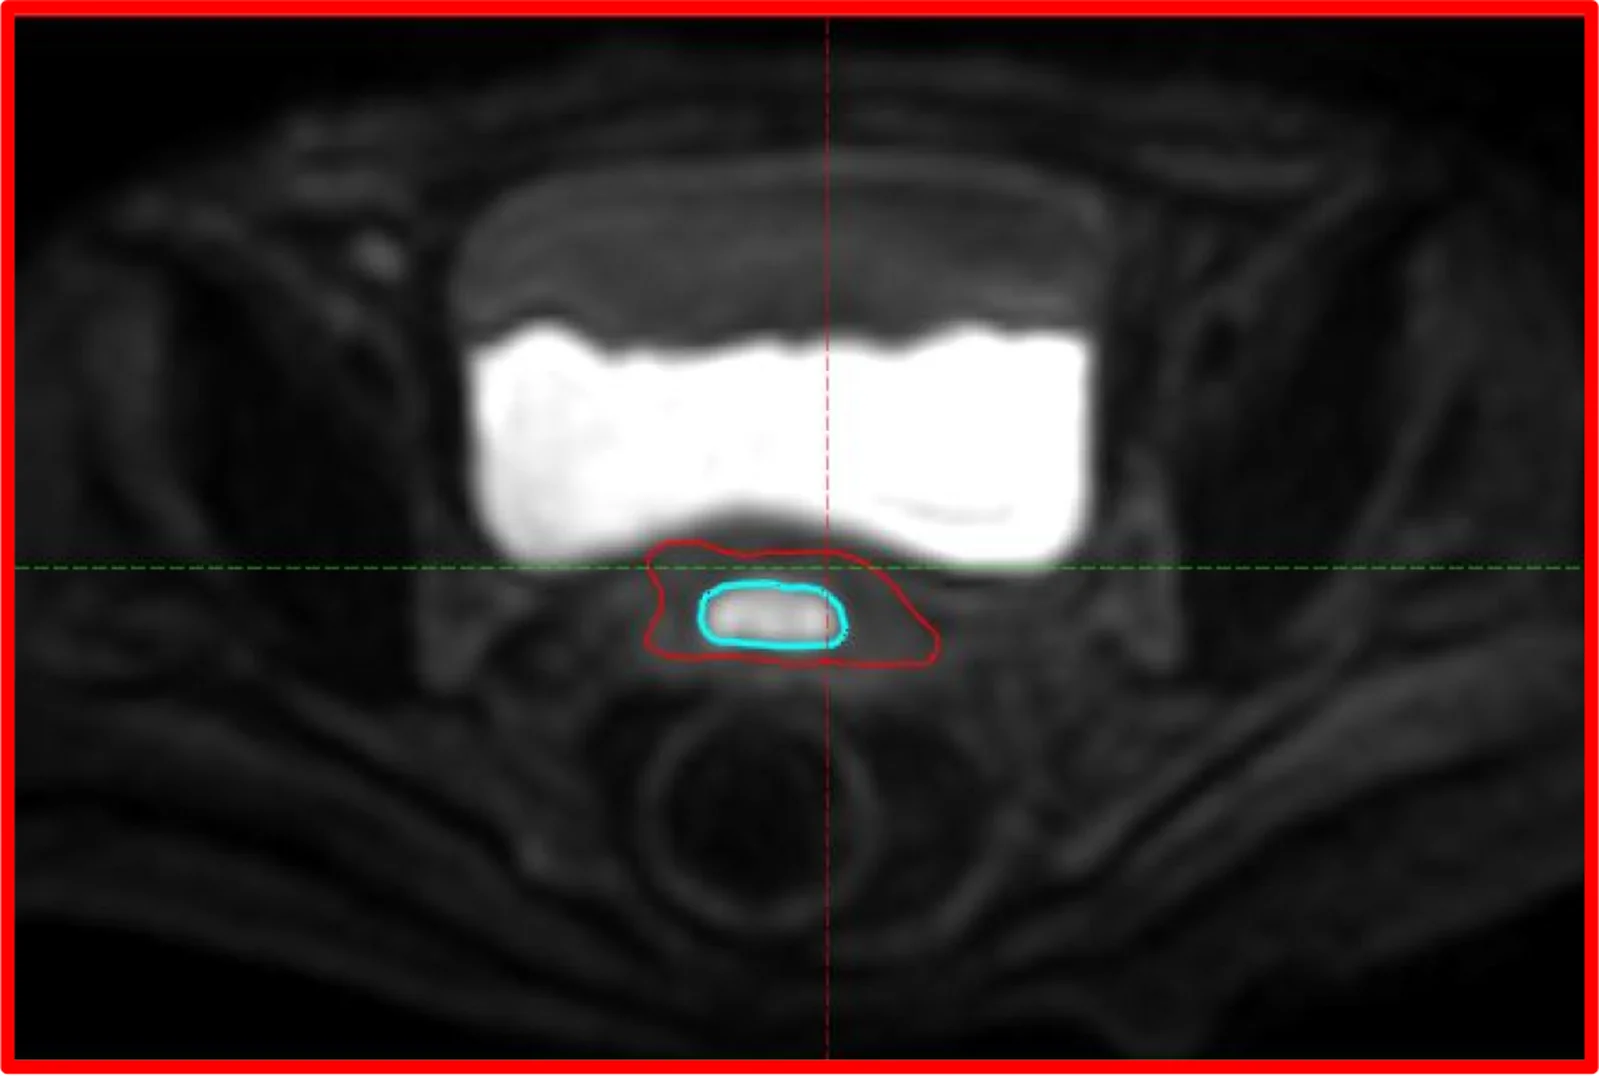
a. Figure showing the GTV at pre_brachytherapy T2 weighted MRI; GTV_T2 in red outline. (For interpretation of the references to colour in this figure legend, the reader is referred to the web version of this article.) b. Figure showing the GTV at pre_brachytherapy on DWI; superimposed GTV_T2 in red outline and GTV_T2/DWI in cyan outline. (For interpretation of the references to colour in this figure legend, the reader is referred to the web version of this article.)
